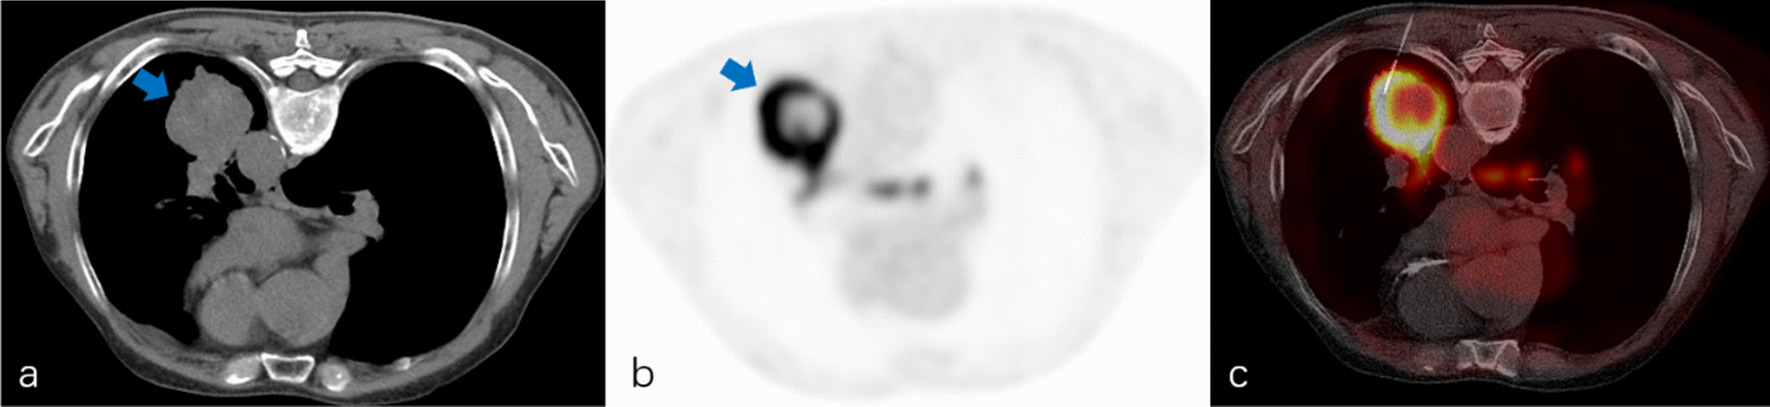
A 69-year-old man with suspected primary lung malignancy. a The intraprocedural non-contrast CT image (using mediastinal soft tissue window) showed a homogenous mass. b
